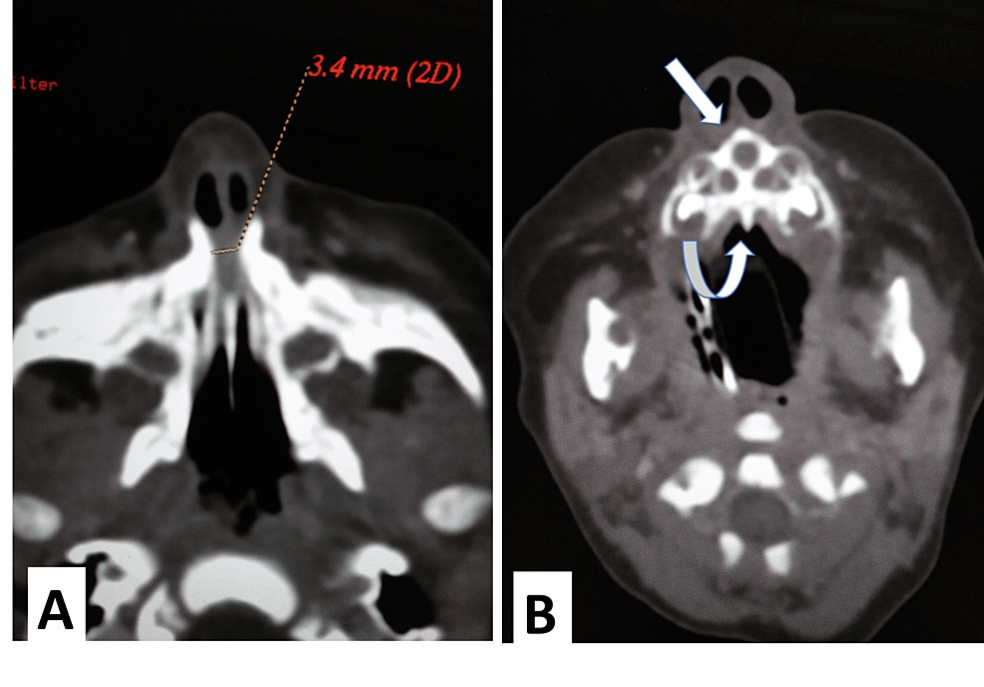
Preoperative maxillofacial CT scan Maxillofacial CT showed (A) a pyriform aperture width of 3.4 mm at the inferior meatus. (B) Single median incisor (straight arrow), median palatal ridge (curved arrow), triangular palate, and patent posterior choana.
